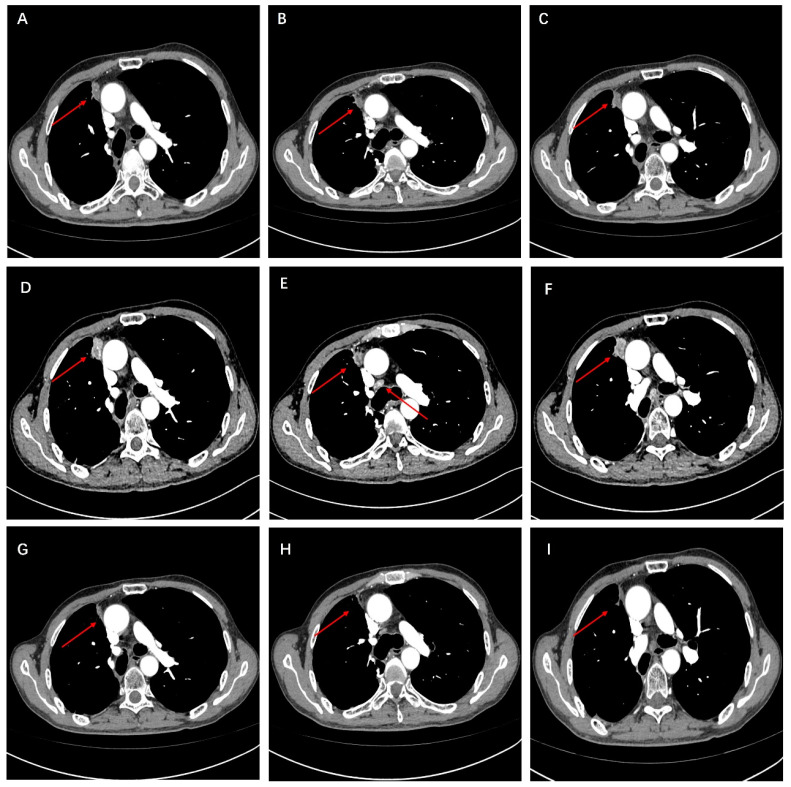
(A–C) At 27 months post-surgery, a follow-up chest-enhanced CT revealed nodules indicative of pleural metastasis on the right side. (D–F) After three months of continued treatment with the original regimen following recurrence, a follow-up CT scan revealed pleural metastatic lesions and enlarged mediastinal lymph nodes. (G–I) After three months of adding bevacizumab to the original regimen, a follow-up CT scan revealed a reduction in pleural metastatic lesions and shrinkage of mediastinal lymph nodes.
